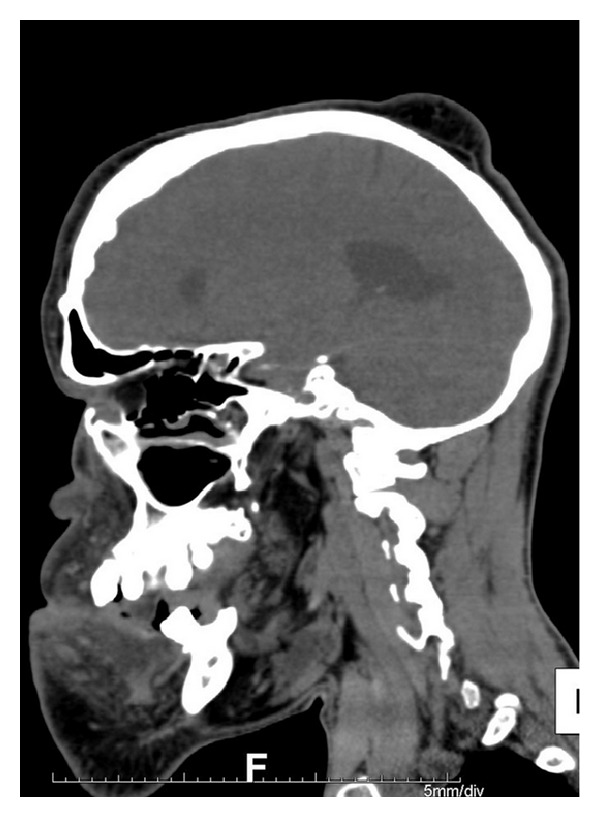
Sagital CT scan showing soft tissue swelling in right high parietal region.
